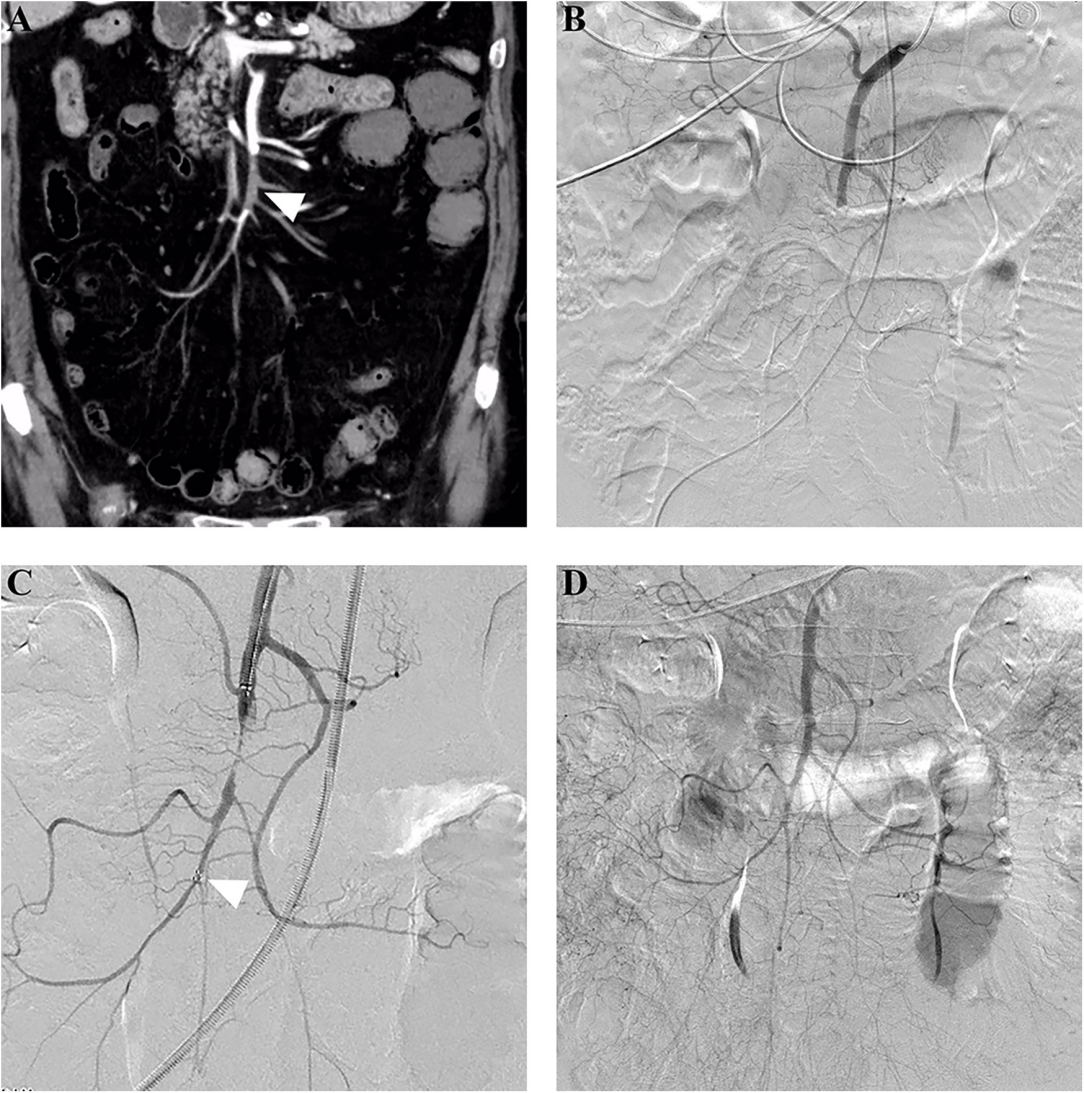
Successful stent thrombectomy in a patient with acute embolic SMA occlusion. (A) An 80-year-old woman presented with acute abdominal pain for 24 h, and subsequent computed tomography angiography revealed embolic occlusion (arrowhead) in the middle SMA stem. (B) Pre-procedure angiography further confirmed the occlusion without distal SMA stem and branch visualization. (C) Stent thrombectomy using a 6 mm × 30 mm Solitaire AB stent was performed. The arrowhead indicated the distal mark of the stent. (D) Post-procedure angiography showed complete SMA revascularization after one thrombectomy attempt. SMA,superior mesenteric artery.
